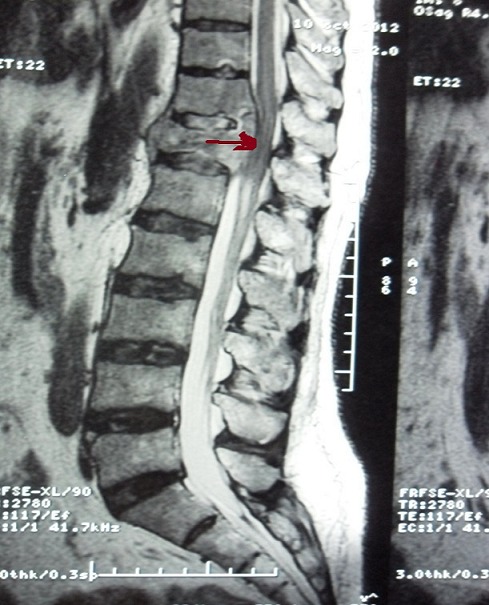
Spinal MRI, sagittal view: spinal cord compression in relation to the twelfth dorsal vertebra
